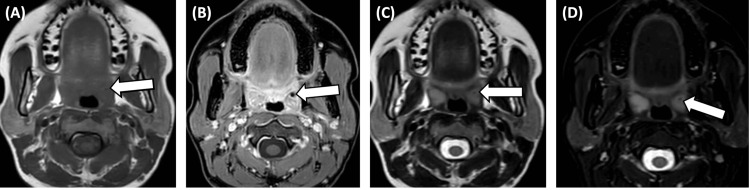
MRI evaluation of the left palatine tonsil. (A) Axial T1-weighted pre-contrast image demonstrating a focal hypointensity in the left palatine tonsil, likely representing post-excision biopsy changes, with no evidence of residual or recurrent mass. The right palatine tonsil and posterior pharyngeal wall appear normal.
